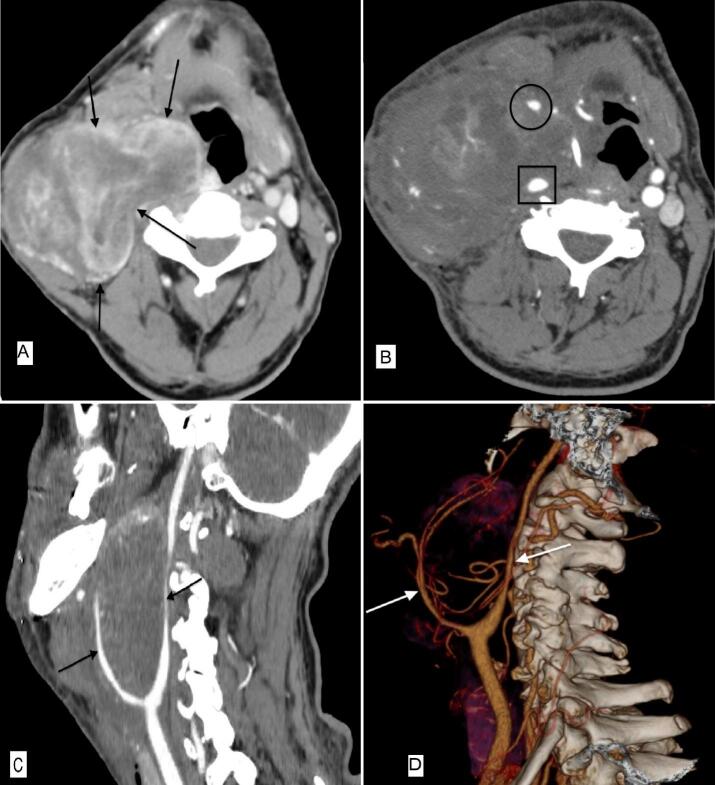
A. Axial contrast-enhanced CT images of the neck demonstrate heterogeneous enhancing well-demarcated mass on the right side with deep extension to the parapharyngeal space and significant compression effect on adjacent structures (arrow). B. Axial CTA of the patient confirms the mass hypervascularity, multiple internal arterial branches, and also splaying of the internal (rectangle) and external (circle) arteries. Sagittal (C) and volume-rendering (D) CTA reconstruction again demonstrate splaying of the internal and external carotid arteries (arrows).
